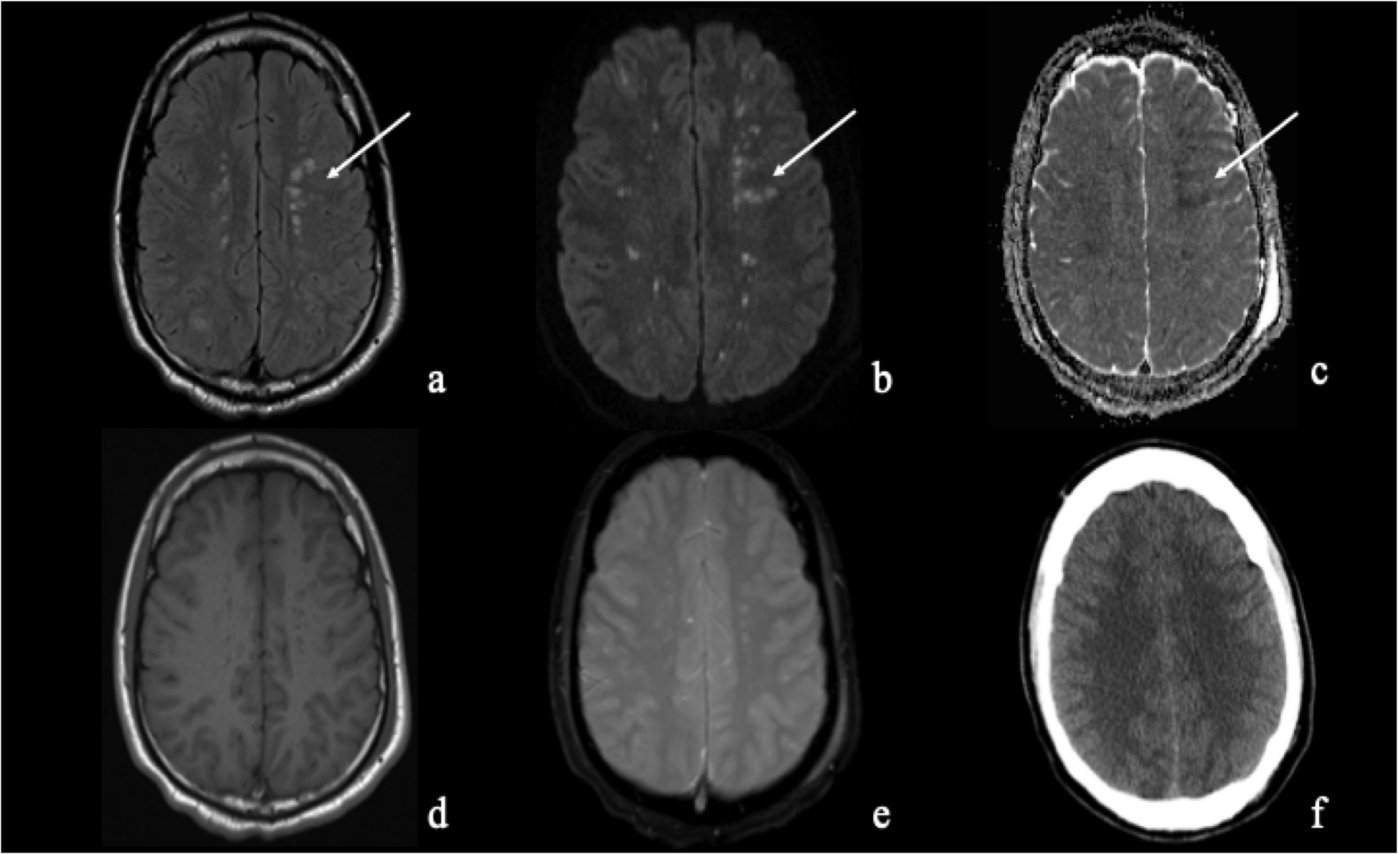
Axial FLAIR (a) magnetic resonance imaging sequence shows the presence of numerous multifocal hyperintensities involving the deep white matter of both hemispheres (arrow), which correspond to innumerable tiny foci of diffusion restrictions on DWI (b) with low signal on ADC map (c) (arrow). These findings are the so-called “starfield” pattern characteristic of cerebral fat embolism syndrome. In the same patient, the SE-T1W (d) and T2* GRE (f) sequences, and the axial non enhanced computed tomography NECT scan (f) appear relatively normal
